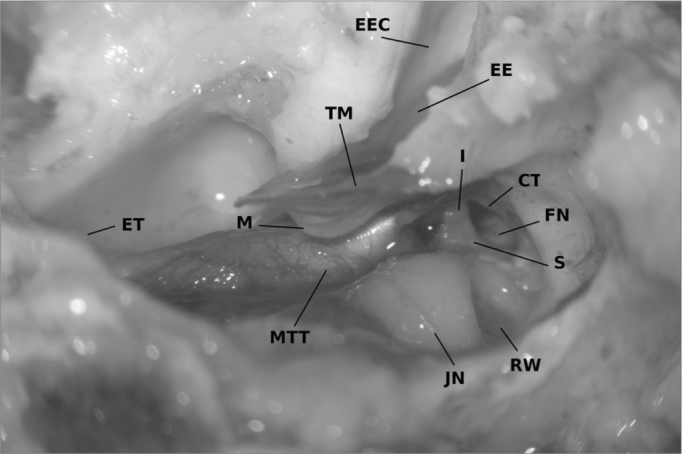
Lambs' middle ear anatomy (right ear) seen from the opened hypotympanon. The external ear canal (EEC) has been opened for measurements and exposes the epitympanal extend (EE) of the tympanic membrane. The tympanic membrane (TM) with the curved malleus' handle (M) can be seen. The tensor tympani muscle (MTT) inserts directly on the handle with the chorda tympani (CT) bending around. The stapes (S) is encircled by the facial nerve (FN) and connected to the long process of the incus (I). RW = round window, JN = Jacobsons' nerve, ET = Opening to the Eustachiian tube
